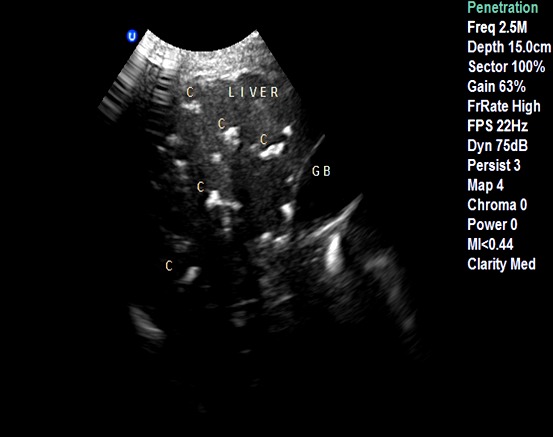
Abdominal ultrasonogram at the level of the liver showing multiple brightly echogenic lesions casting posterior acoustic shadows in the liver parenchyma, distorting its architecture
